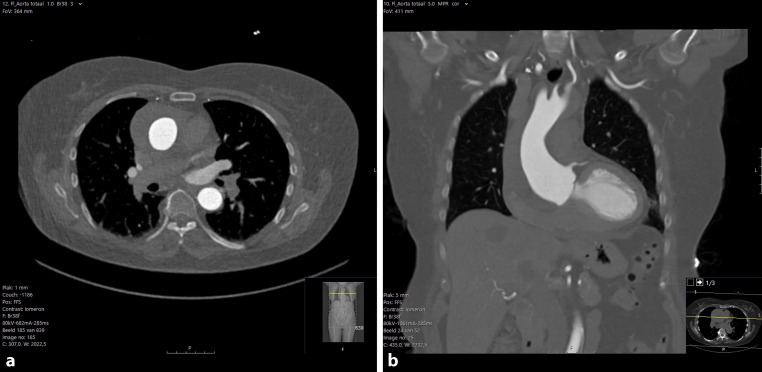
a, b CT-scan of the aorta after symptom onset showing an intramural hematoma located at the aortic root and ascending aorta
